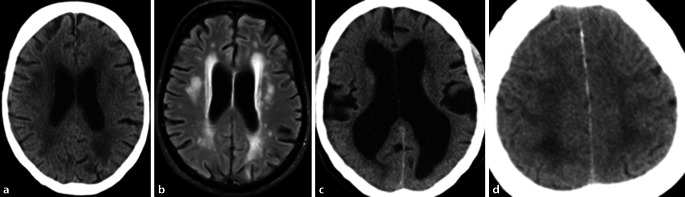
Axial sections showning signs of subcortical hypodensities (CCT, a) or hyperintensities (MRI, b) as manifestation of atherosclerotic encephalopathy (SAE) leading to impairment of motor function and cognition in a 78-year-old patient with diabetes mellitus and hypertension. Signs of normal pressure hydrocephalus (NPH) with dilatation of ventricles (c) and tight sulci in the high frontoparietal regions (d) as a typical geriatric syndrome with gait disturbances, falls, impaired cognition and urinary incontinence
